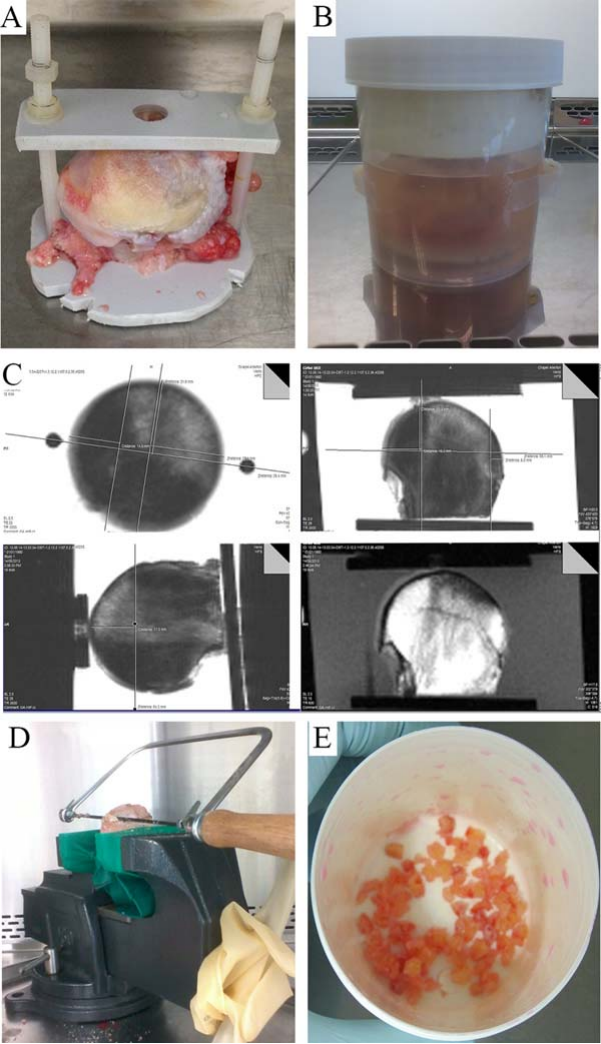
Segregation of bone marrow lesion (BML) and non‐BML regions and downstream processing. A, Femoral head in plastic bracket. B, Femoral head/bracket in a phosphate buffered saline–containing polypropylene jar. C, Proton density–weighted sequence magnetic resonance images in 3 planes, with measurements for cuts (top and bottom left) and corresponding T1‐weighted sequence image obtained in the “coronal” plane (bottom right). D, Bone segregation apparatus. E, BML bone fragments used for collagenase treatment after mincing with a rongeur.
